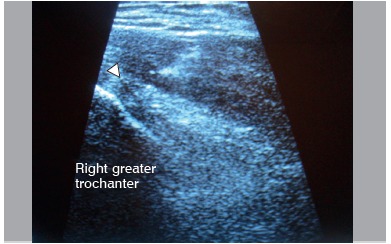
Coronal ultrasound view at the time of infiltration. The arrow indicates the needle at the time of infiltration.
